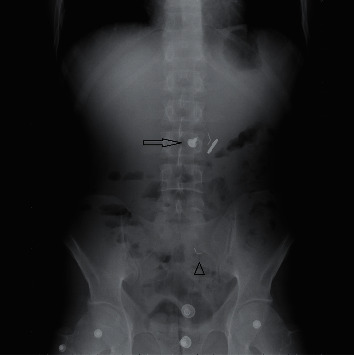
Abdominal X-ray: arrow showing 3 metallic objects (screw, coin, and plastic head pin) in the stomach, and arrow head showing another plastic head pin well into the small intestine. Note that the small metal scrap that was later seen on endoscopy is not evident here.
